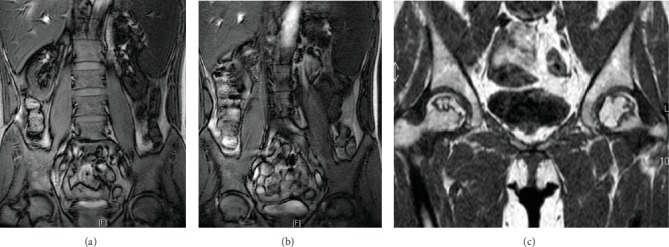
A 56-year-old male developed ONFH after DBRs treatment for 76.4 months. (a, b) Coronal magnetic resonance image before DBRs. (c) Coronal magnetic resonance image after cumulative dose of 4000 mg dexamethasone for 76.4 months. ONFH: osteonecrosis of femoral head; DBRs: dexamethasone-based regimens.
